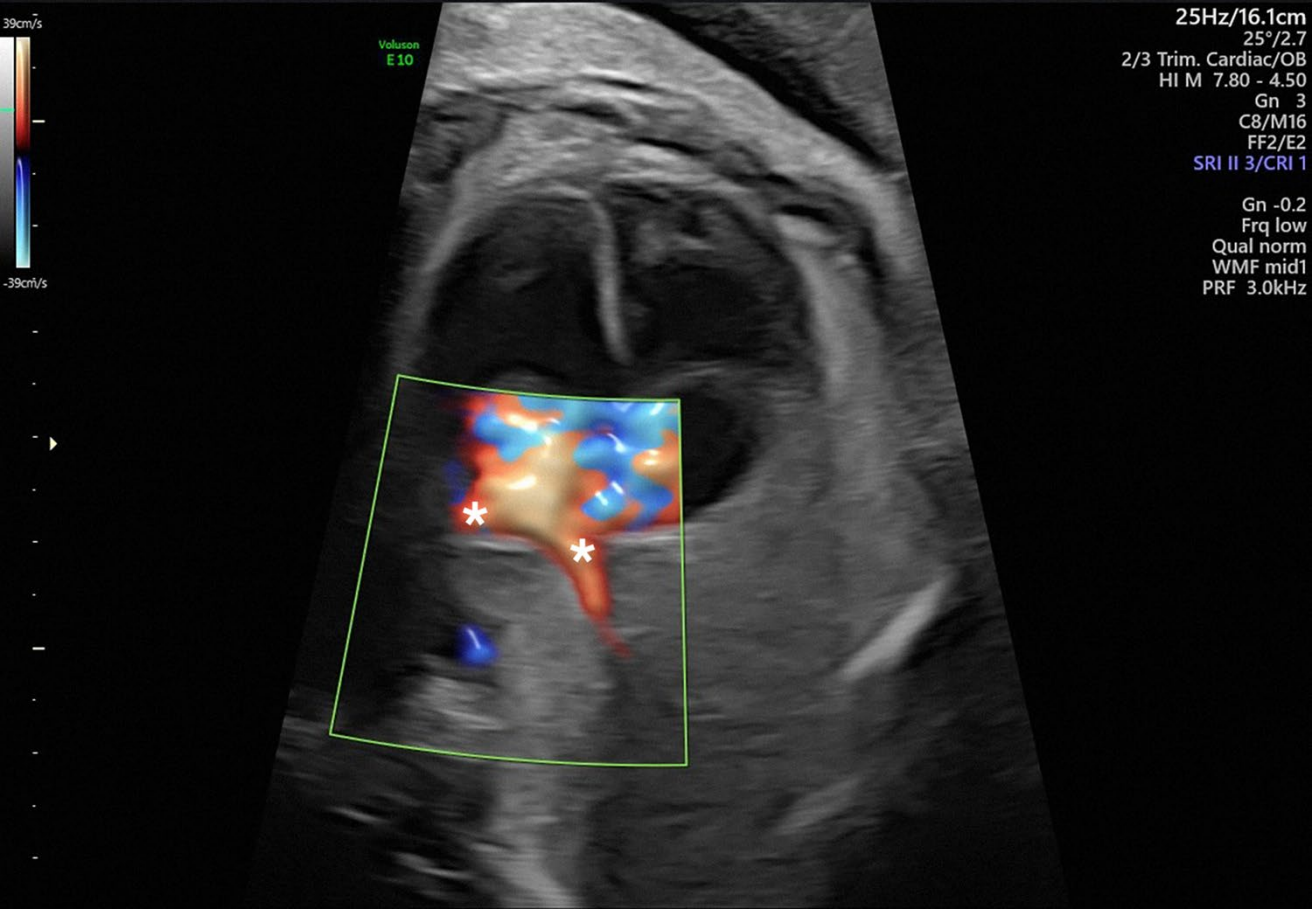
Color Doppler in fetal echocardiography: applying color Doppler can help in the identification of the pulmonary veins (*)
